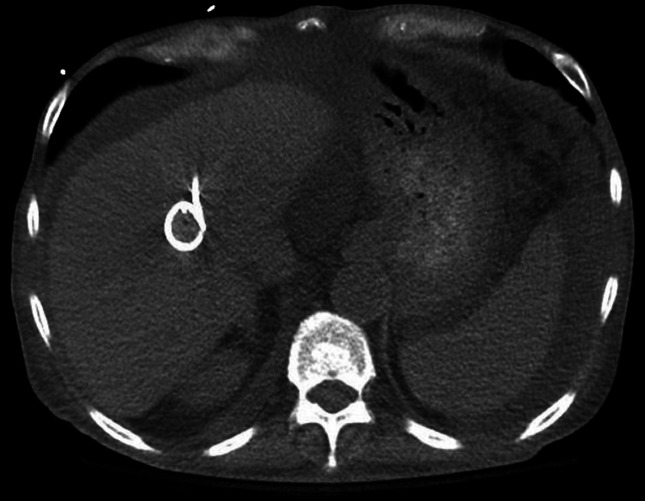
CT scan of the liver abscess with the drain in place.
